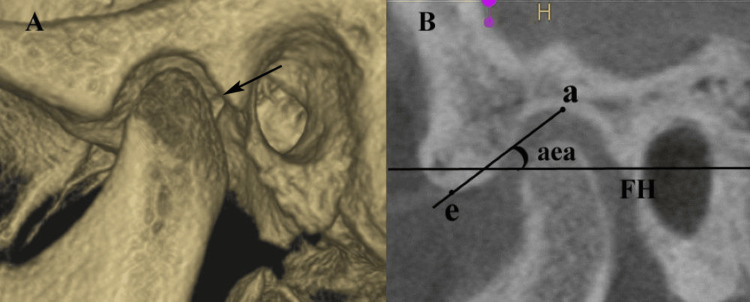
(A) CBCT image of temporomandibular joint and (B) articular eminence inclination angle (aea). This figure represents CBCT images of a study patient, included with appropriate informed consent. a: highest point on temporal fossa; e: highest point on articular eminence; FH: Frankfort Horizontal plane; CBCT: cone-beam computed tomography (sagittal view)
